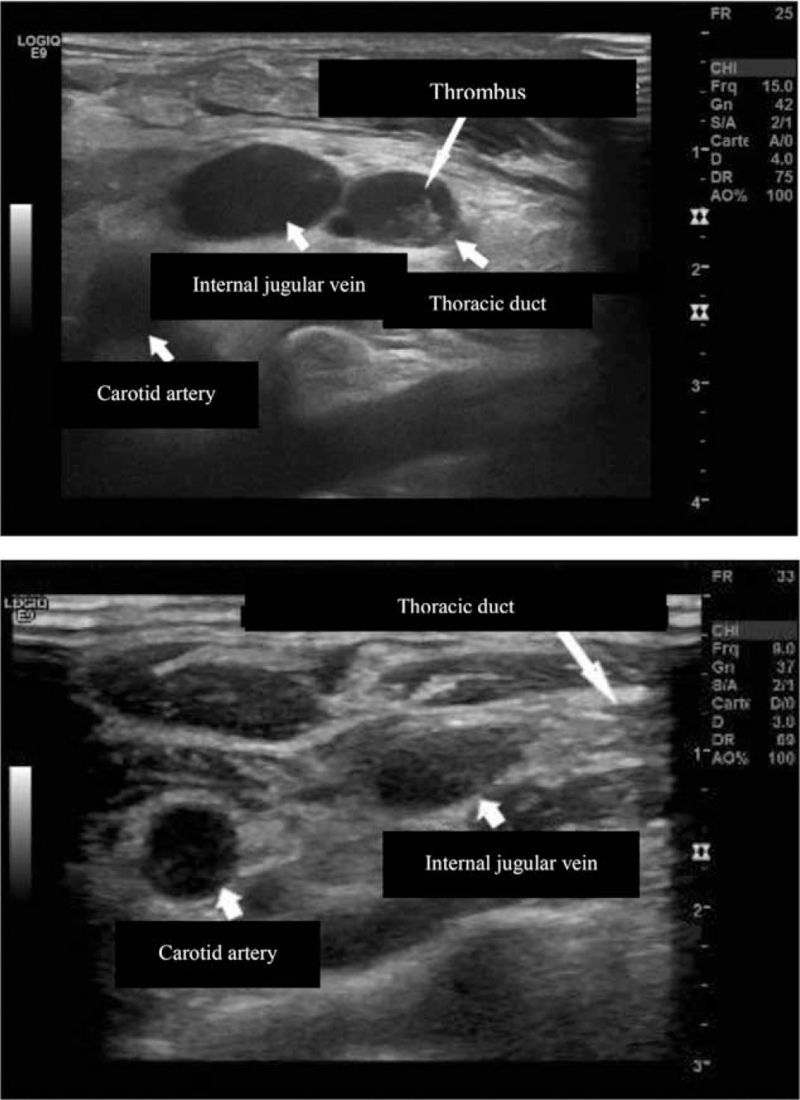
Ultrasonography sections of a patient in observation 1 (top) versus a healthy patient: dilated thoracic duct with holding an echogenic structure (thrombosis).
